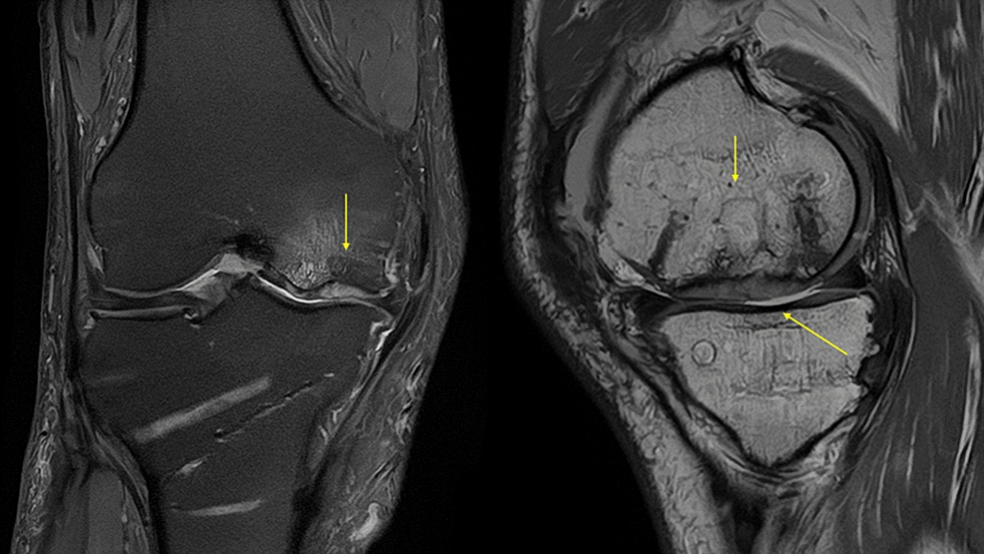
MRI at one year postoperatively The arrows indicate lesions with autologous chondrocyte implantation.
